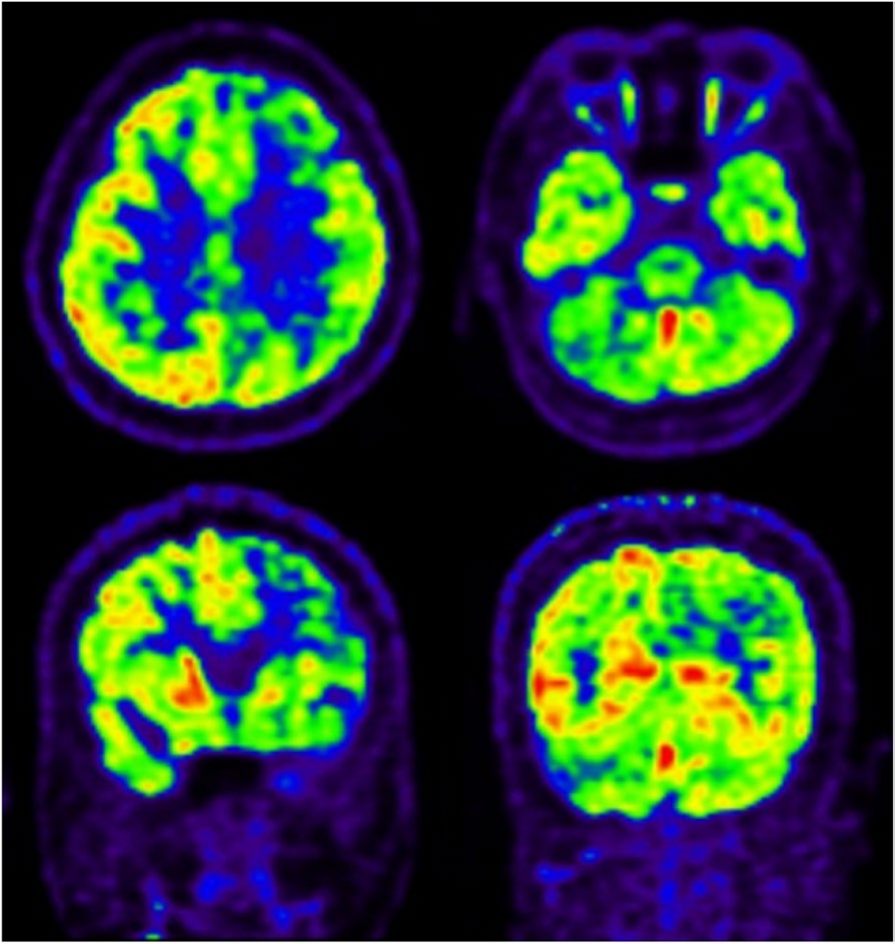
PET-CT of the patient on 10–2020, showed diffuse decreased metabolic heterogeneity in the bilateral cerebral hemispheres, mainly in the left frontal lobe, and a diffuse decrease in metabolism in the right cerebellum, which was considered CCD
